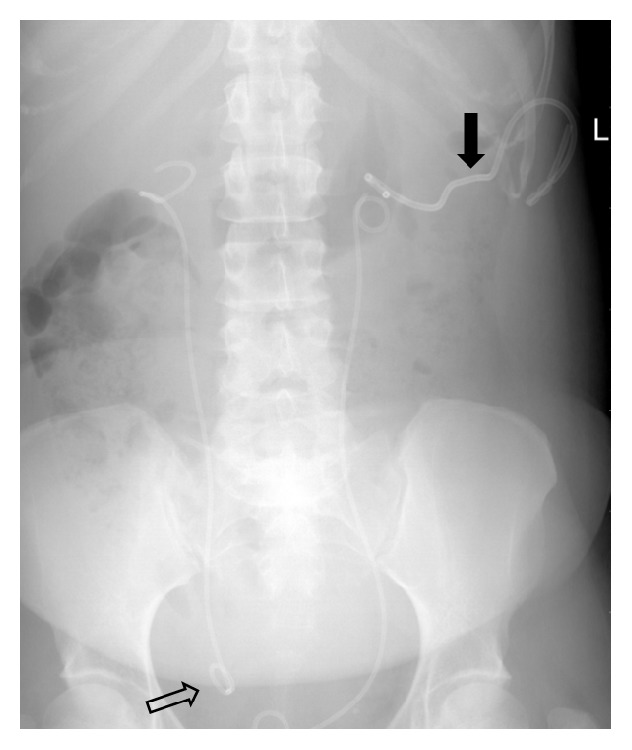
X-ray KUB following failed removal of encrusted left sided percutaneous nephrostomy tube (solid arrow) and right sided ureteric stent, which is displaced with the distal end lying in the distal ureter (hollow arrow).
